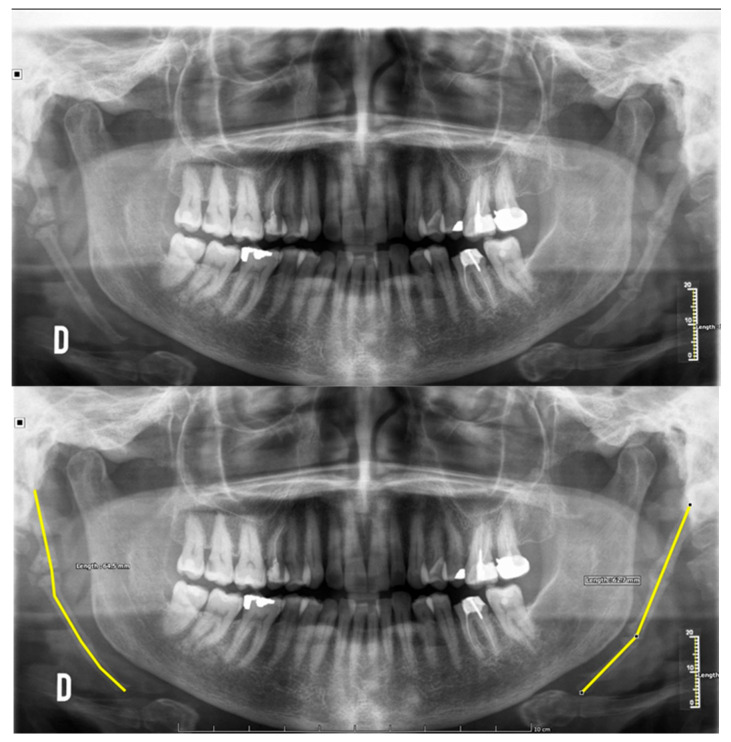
Panoramic radiographs showing elongated styloid processes.
